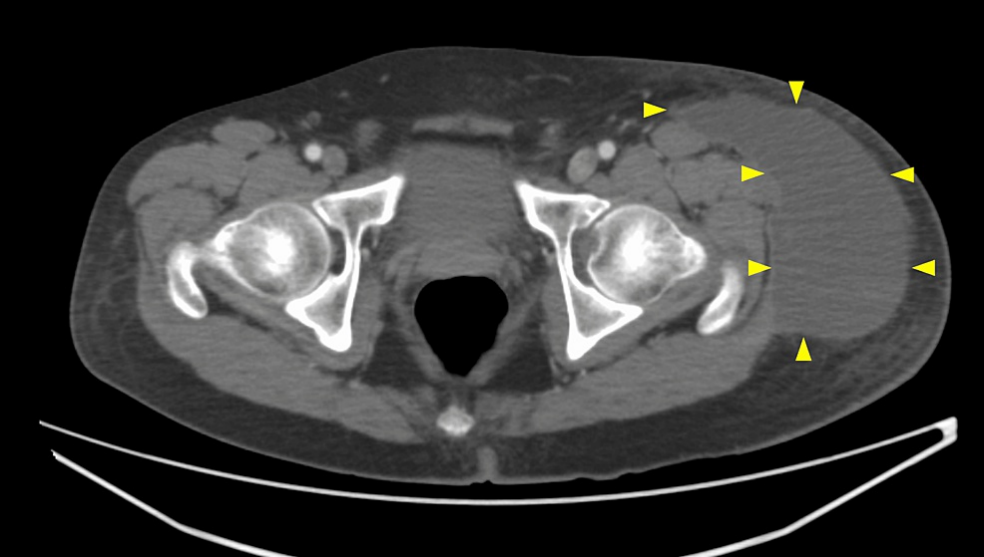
A CT image axial plane A hematoma-like effusion was observed on the lateral aspect of the iliotibial band (yellow arrowheads)
